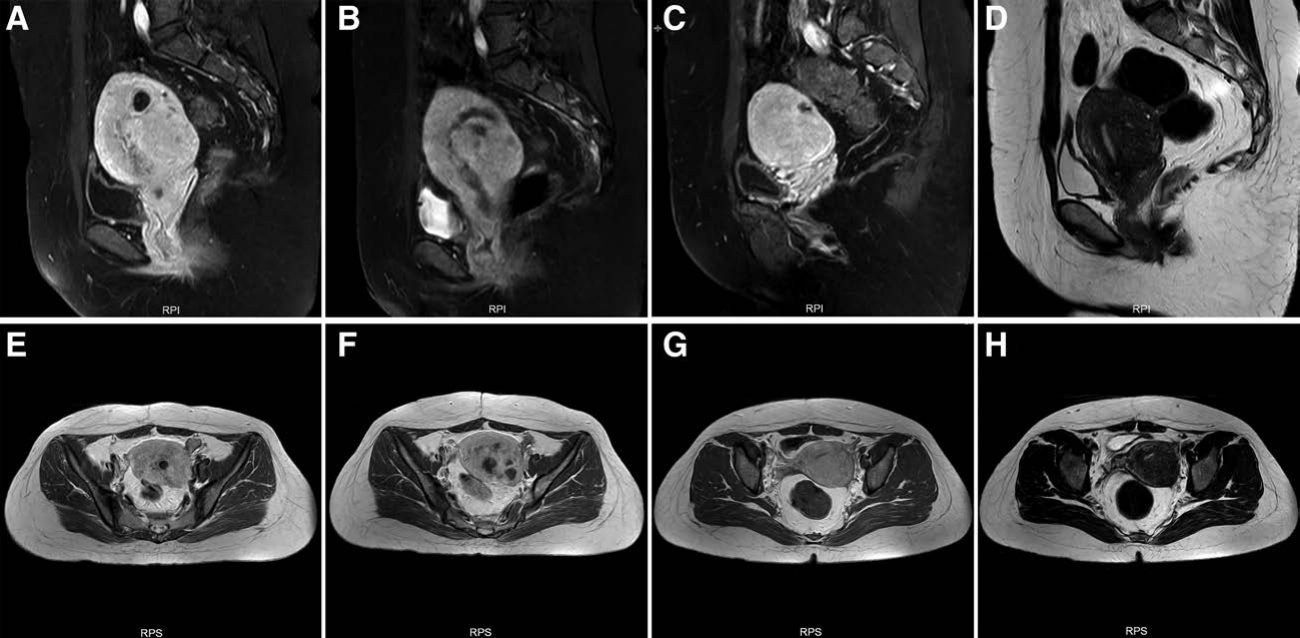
MR image obtained from the adenomyosis patient with IMP. (A) Sagittal and (B) transverse views of preoperative T1-weighed contrast enhanced images showing adenomyosis and intramural pregnancy sac lesions in the posterior wall of the uterus. (C/E) Sagittal and (D/F) transverse views of postoperative T1-weighed contrast enhanced images showing the nonperfused region at the posterior wall of the uterus at post-HIFU (C/D) and 4 months after HIFU (E/F). (G) Sagittal and (H) transverse views of postoperative T2-weighed images only showing adenomyosis lesions in the posterior wall of the uterus 4 months after HIFU. HIFU = high-intensity focused ultrasound, IMP = intramural ectopic pregnancy.
